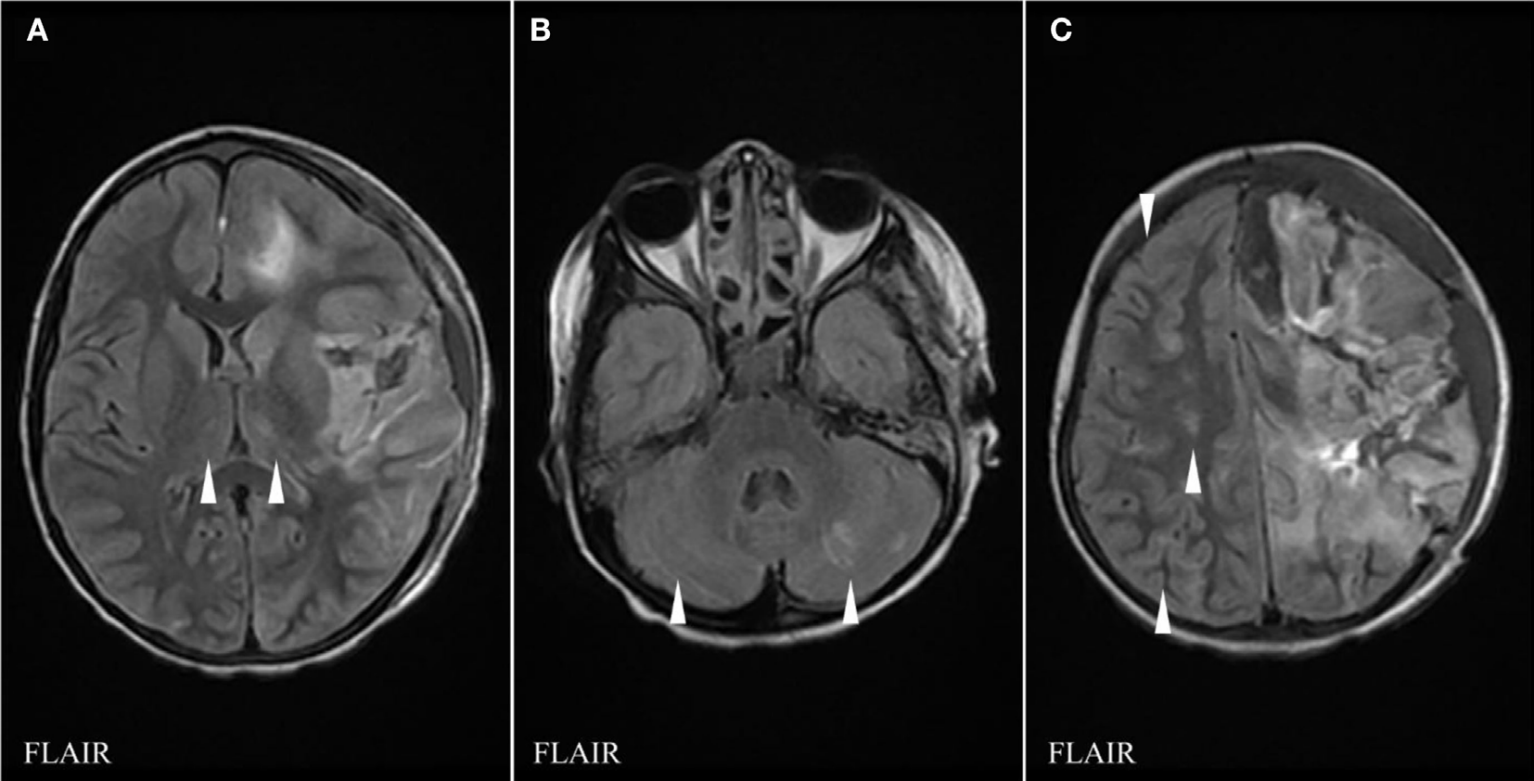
FLAIR sequences of brain magnetic resonance imaging (MRI) at day 39 showing interval evolution and improvement of thalamic (A), cerebellar (B), and right cerebral hemispheric lesions (C) with no additional lesions as compared to the previous MRI (arrowheads indicate hypersignal abnormalities).
